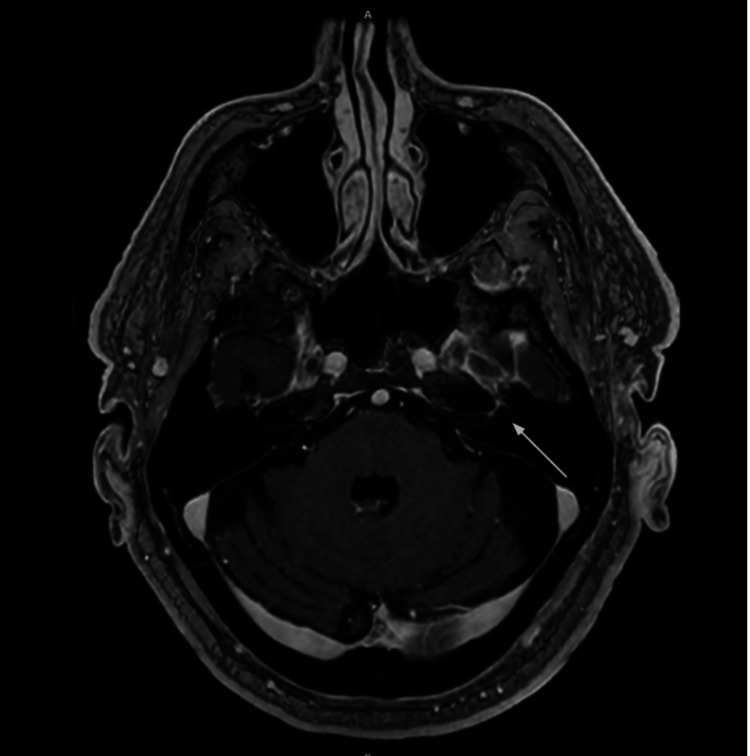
Axial post-contrast MRI demonstrating abnormal enhancement of the left facial nerve (cranial nerve VII) within the labyrinthine segment.
